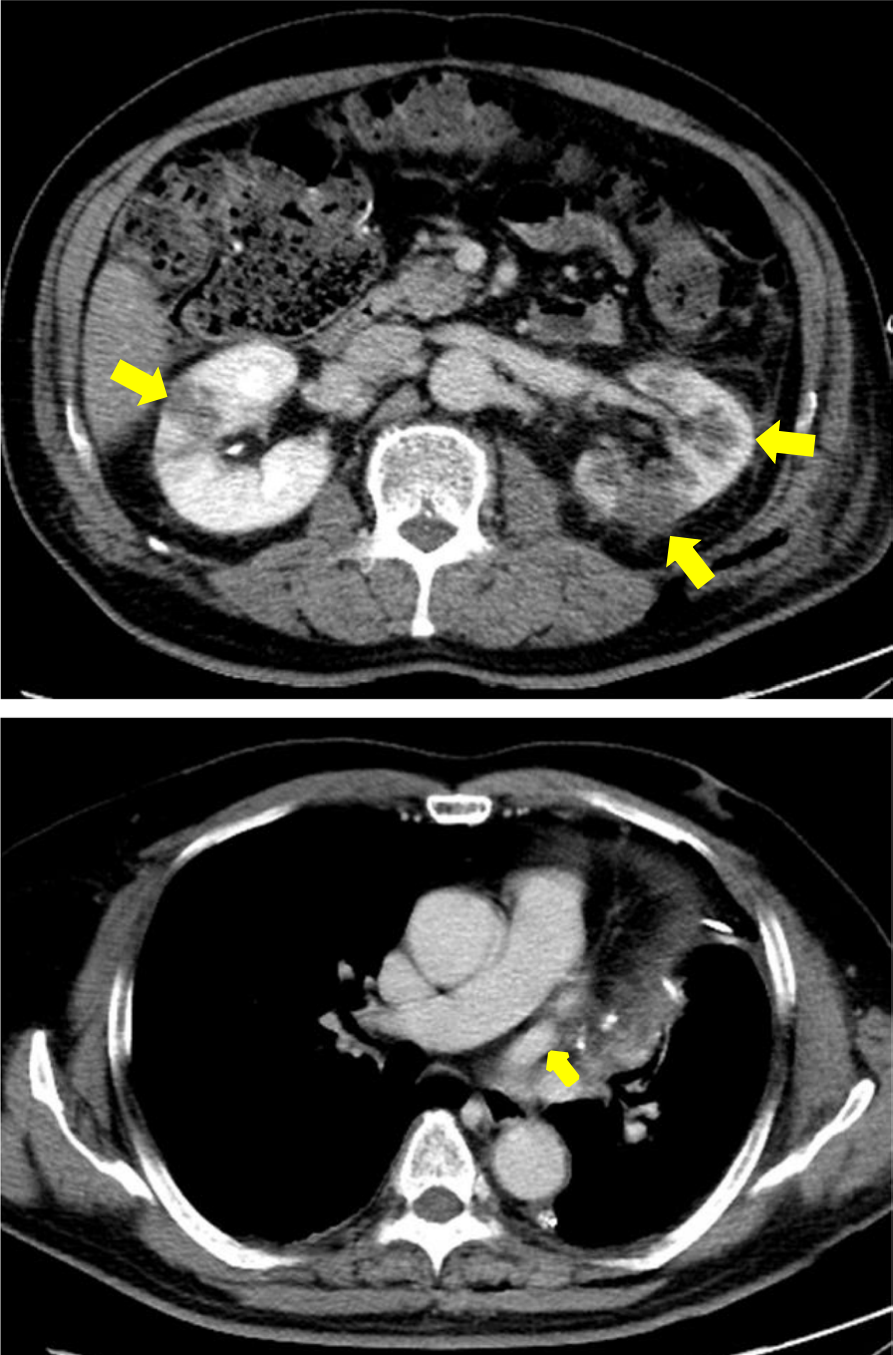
Contrast-enhanced computed tomography showed partial defect of the bilateral kidney and no PVS thrombosis
